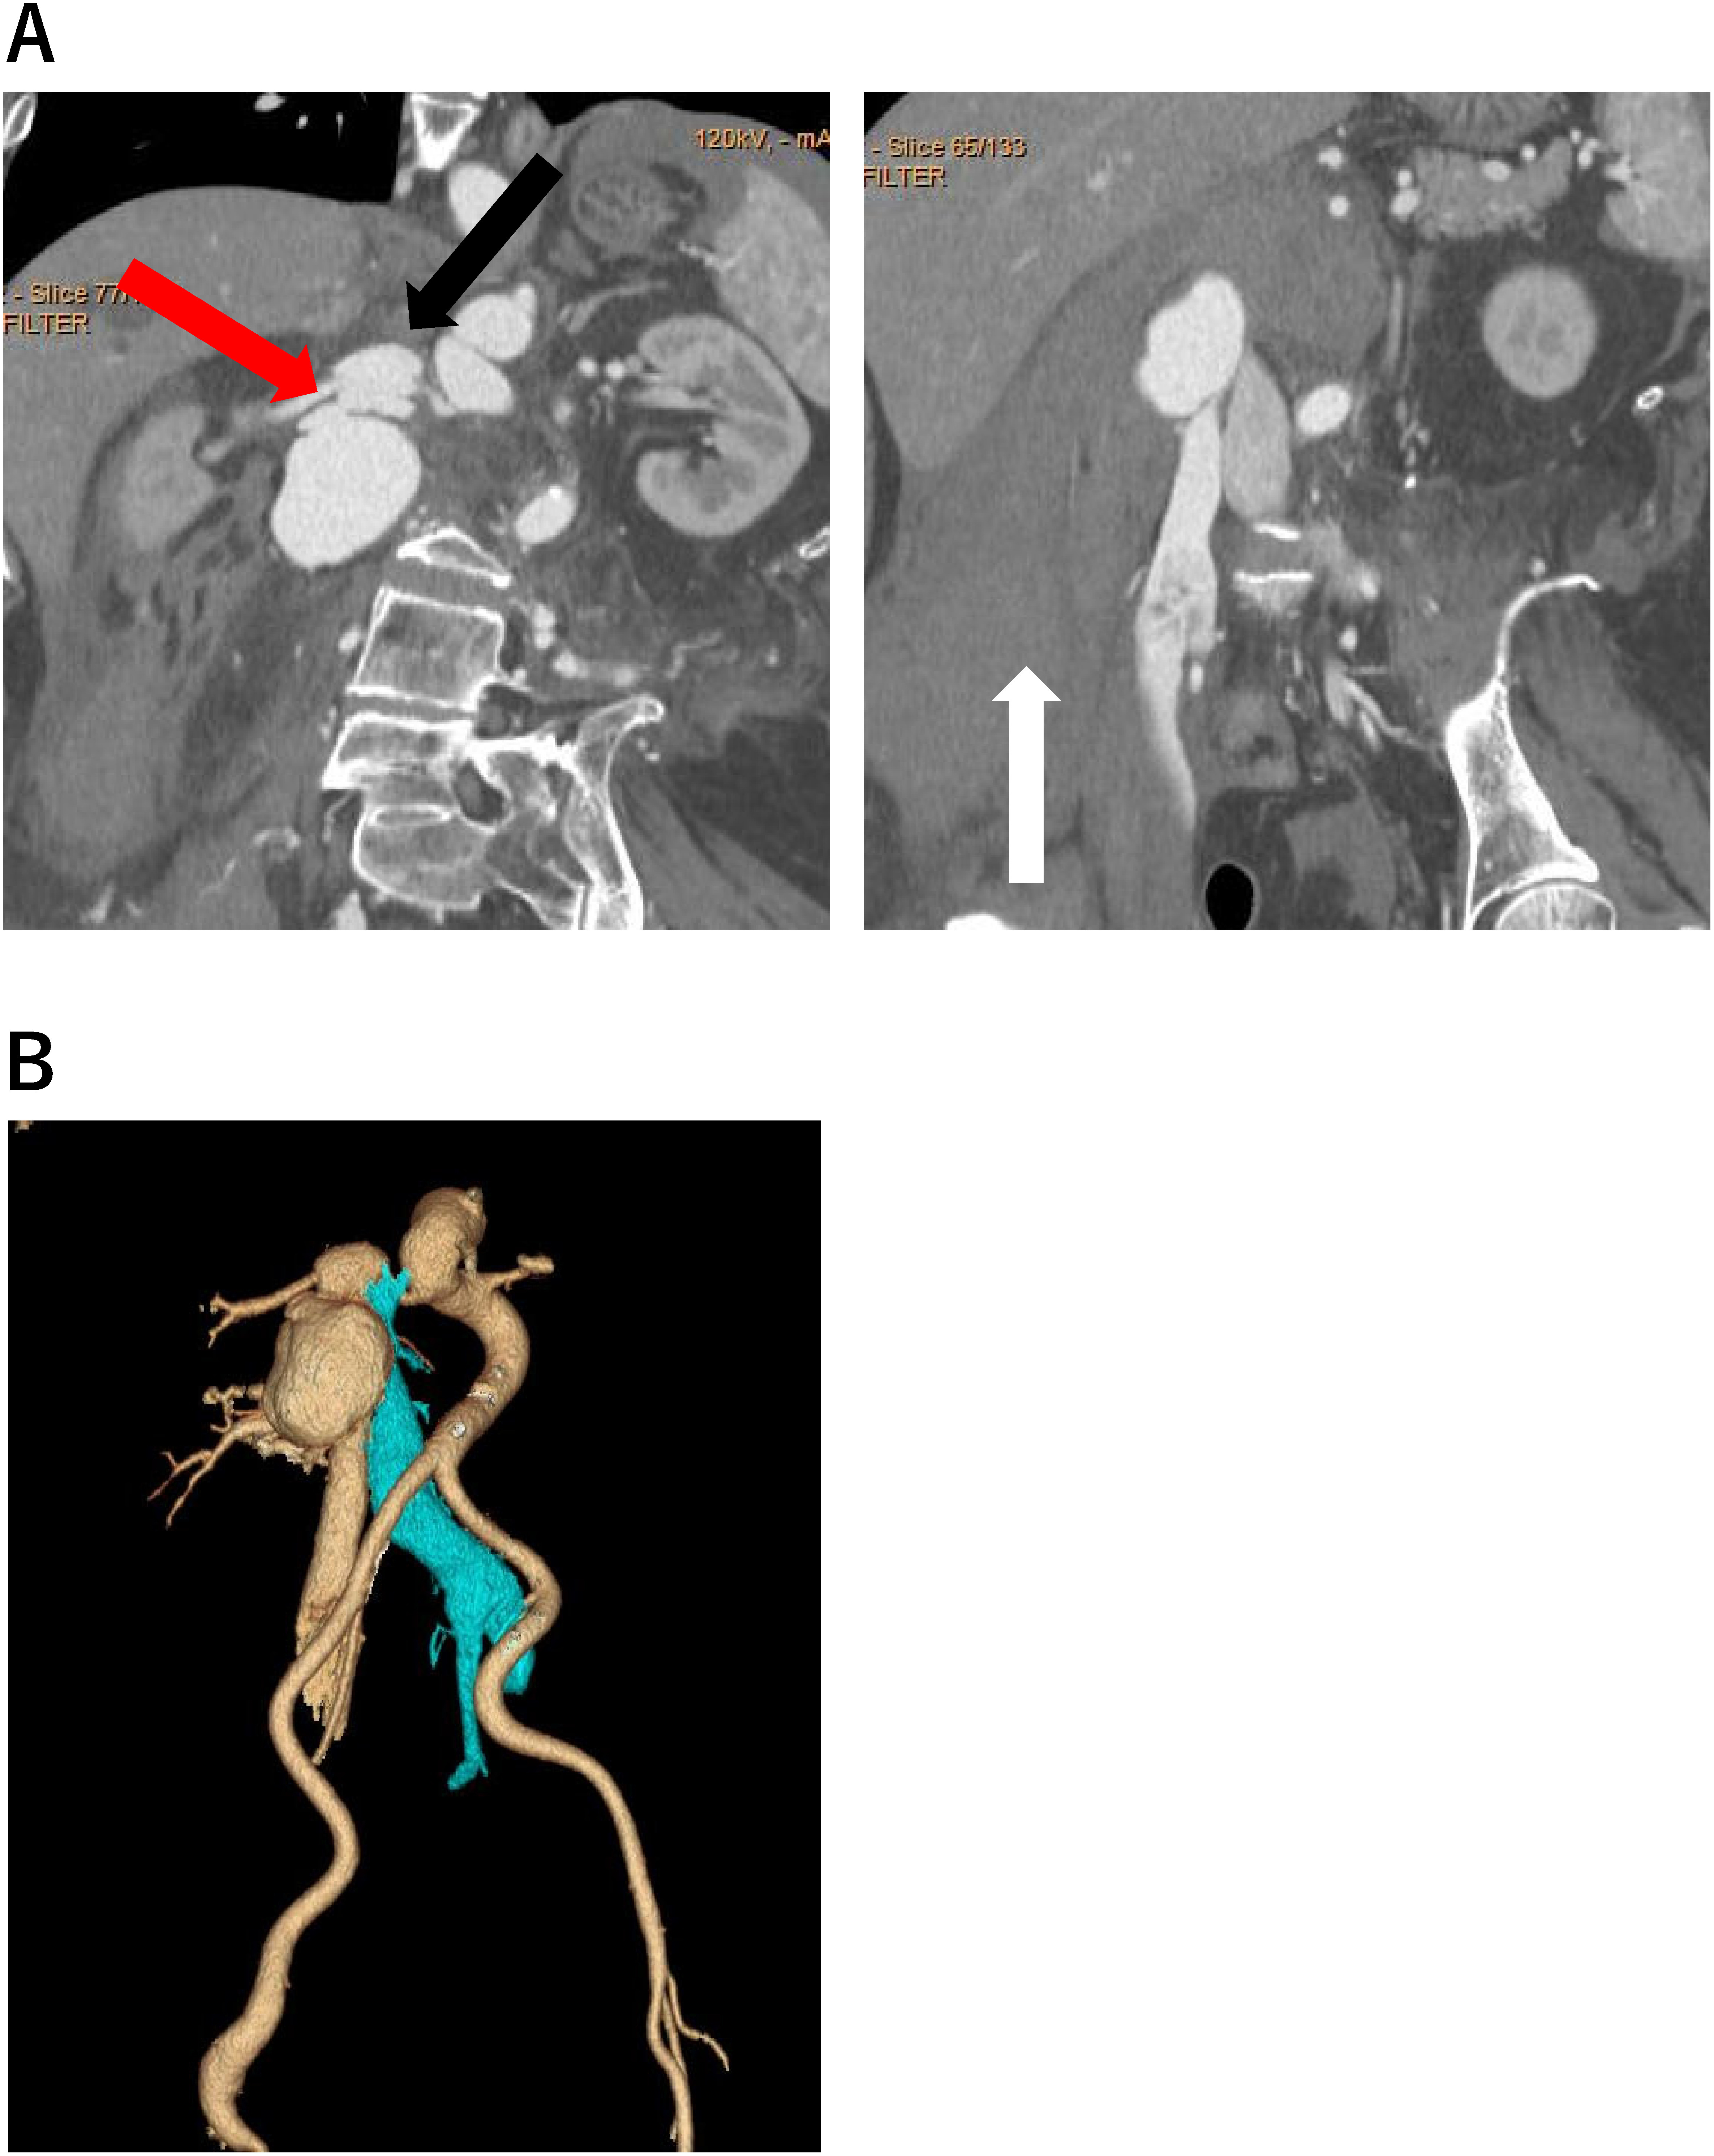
Fig. 1 Preoperative contrast-enhanced computed tomography (CT). (A) Enhanced CT showing a right renal artery aneurysm (black arrow), fistula formation to the inferior vena cava (red arrow), and a large hematoma in the right retroperitoneal space (white arrow). (B) A three-dimensional CT image.
